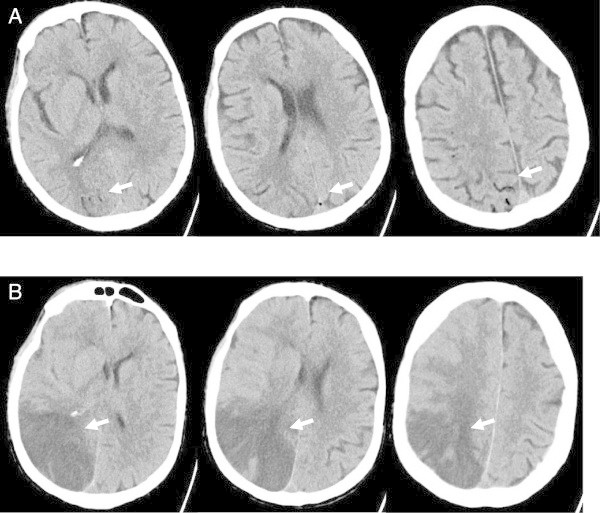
CT in cerebral air embolism via ERCP. Panel A, native axial CT-scan of the brain shows a small amount of air accumulation in the right posterior subarachnoidal space (arrow) and in the superior sagittal venous sinus (arrow). Panel B, native axial CT-scan of the brain two days later shows severe infarction (arrow) predominantly in the right posterior hemisphere with malignant brain swelling.
